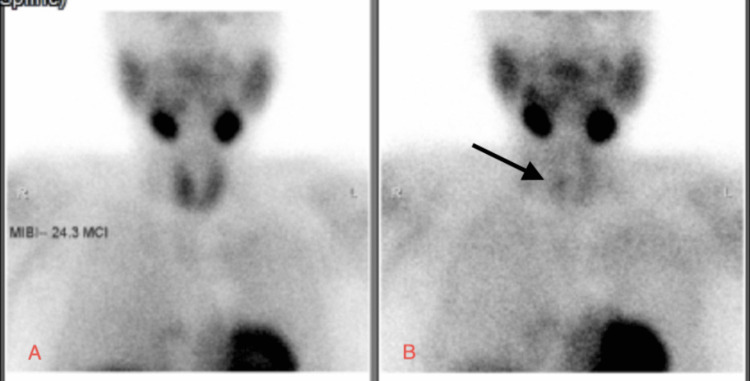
Early (A) and delayed (B) planar images from parathyroid scintigraphy demonstrating a small solitary focus of radiotracer uptake on delayed imaging (arrow), located at the mid-pole region of the right lobe of the thyroid gland, suggestive of a parathyroid adenoma.
